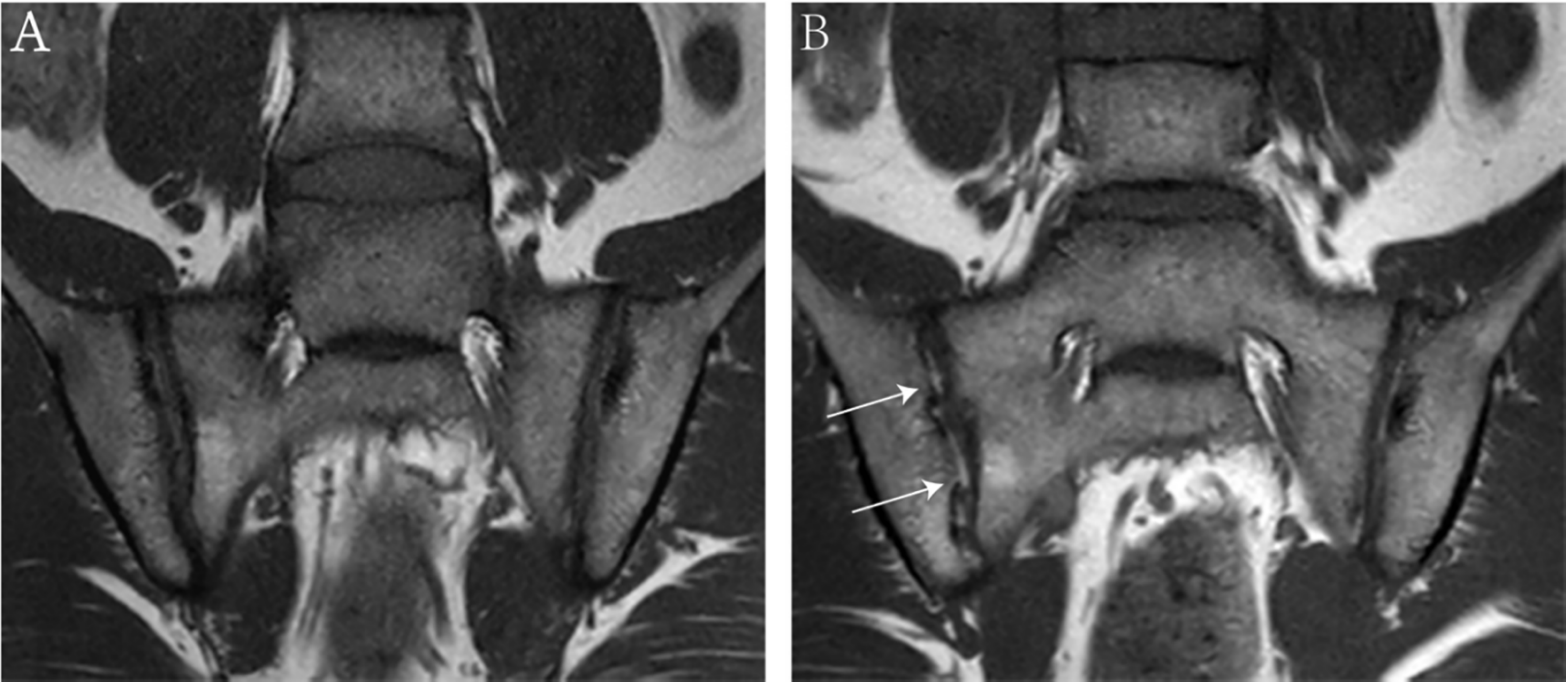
MRI findings of an 18-year-old male with HLA-B27 positive ankylosing spondylitis at baseline (A) and 6-month follow-up (B). Coronal oblique T1 images show new ‘backfill’ at the right sacroiliac joints (white arrows) after 6-month follow-up.
